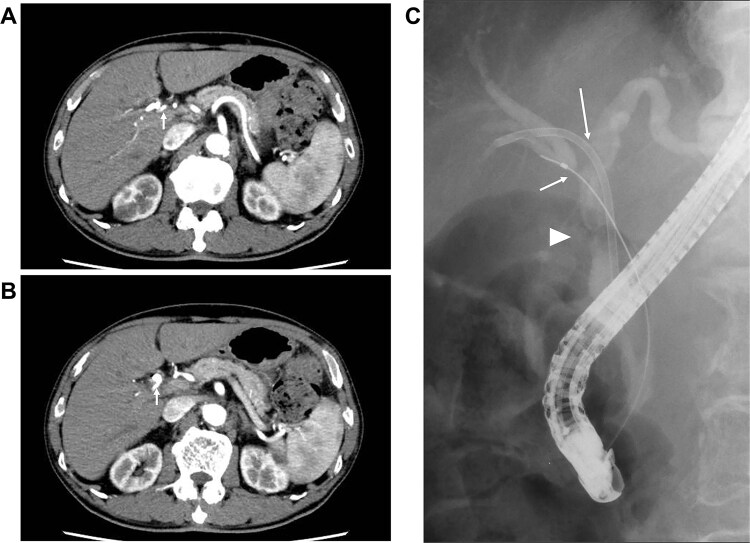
Images after seven cycles of GCD immunochemotherapy (A and B) dynamic CT demonstrating the tumor has shrunk and that the infiltration into the RHA has disappeared (arrow). The enlarged lymph node has also disappeared. (C) ERC revealing a significantly improved CHD stricture (arrow head); however, the stricture at the confluence of the anterior and posterior branches persists (arrow).
